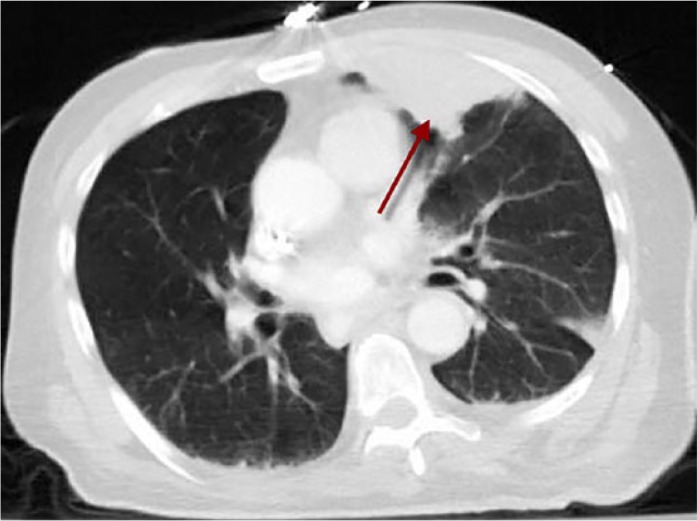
Computed tomography scan of the chest showing a 7.0 × 3.2 cm^2^ pleural-based left upper lobe soft-tissue mass.
